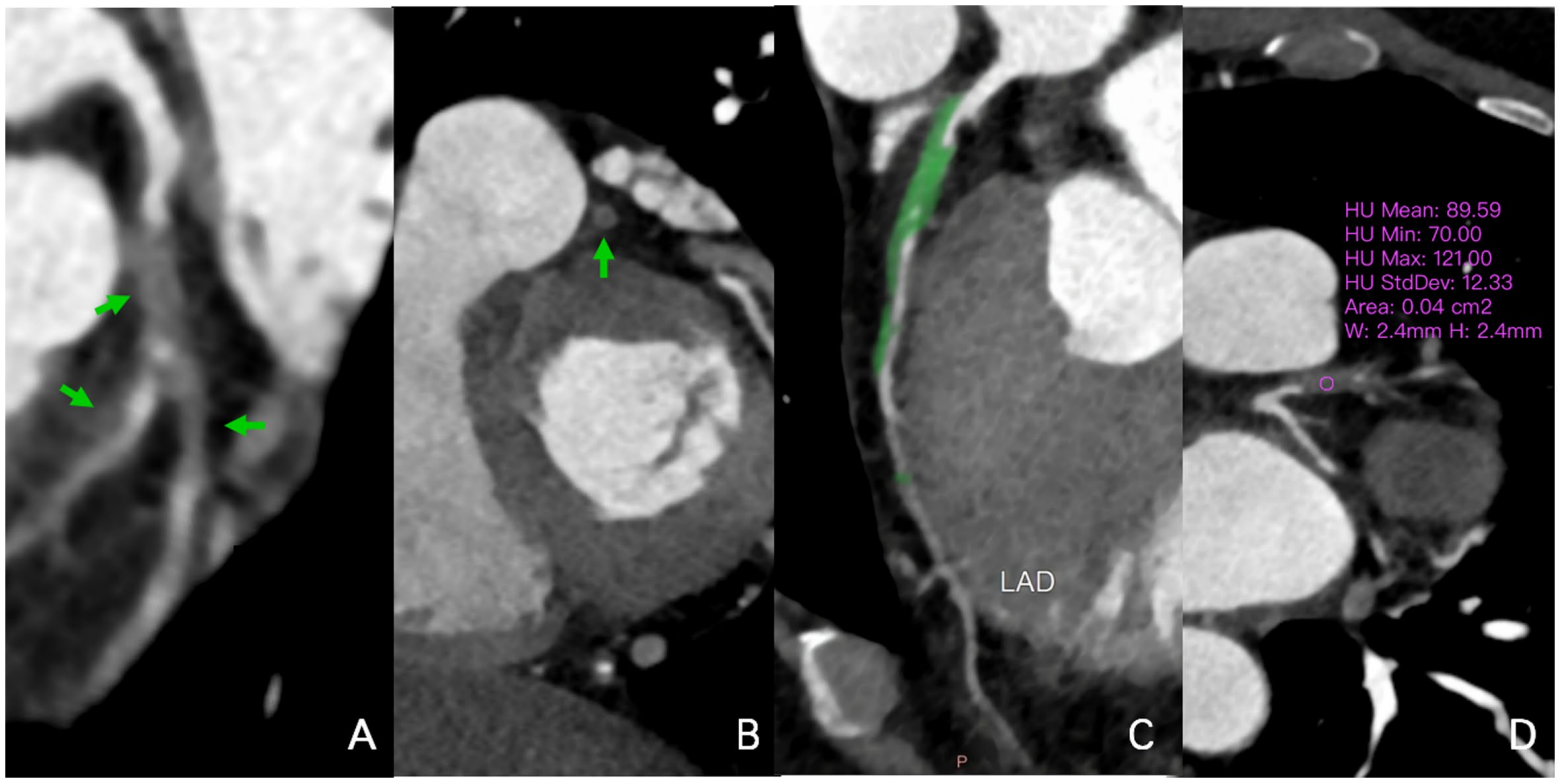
A 58-year-old man infected with HIV. In the multiplanar reconstruction of coronary CTA (A–D), non-calcified plaques (70-121HU) were seen in the proximal segment of the LAD and the beginning of the first diagonal branch, with severe lumen stenosis (90–99%). Noncalcified plaque also was seen in the middle segment of the LAD with mild lumen stenosis (40–50%). HIV, human immunodeficiency virus; CTA, CT angiography; HU, Hounsfield units; LAD, left anterior descending artery.
